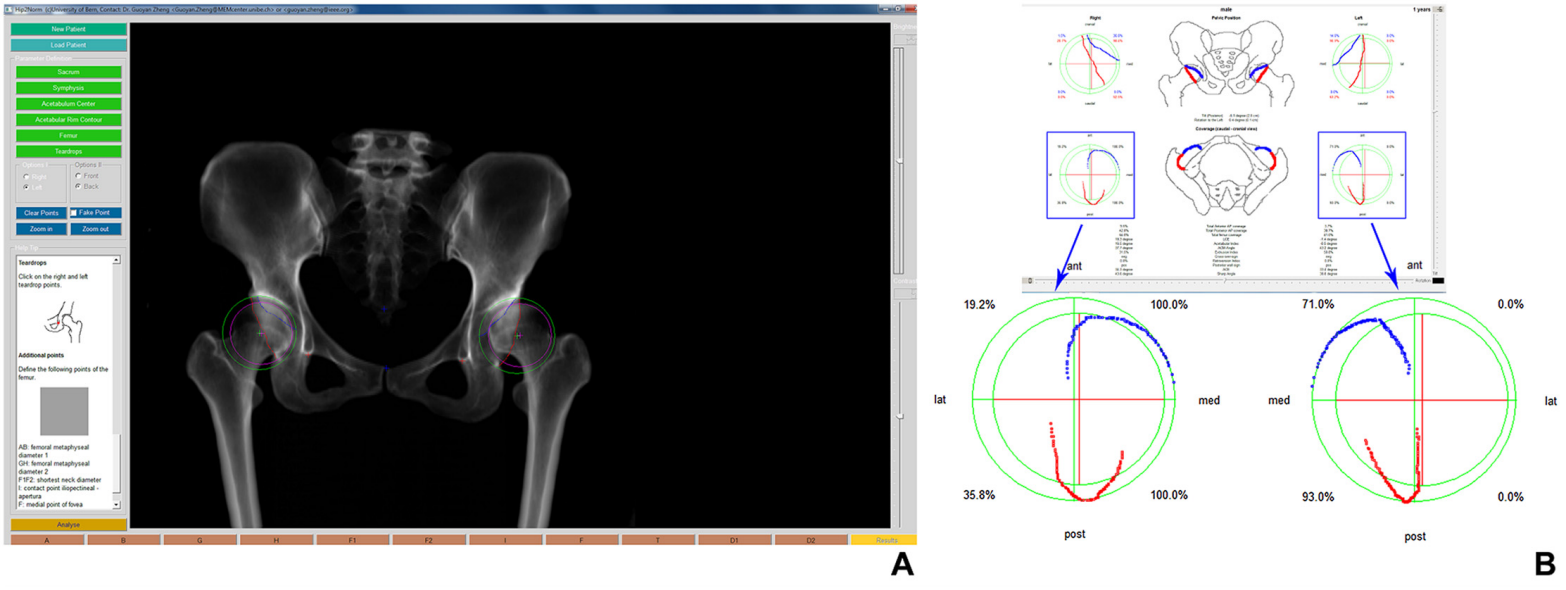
Hip^2^Norm Software. (A) Graphical user interface (left image) for digitizing landmarks for computerized evaluation of an AP radiograph of the pelvis. The inferior margins of the teardrops (red crosses), the outline of the projected anterior and posterior acetabular rim (blue and red line), and the middle of the sacrococcygeal joint (upper blue cross) and the upper border of the symphysis (lower blue cross) must be drawn manually, while the center and the radius of femoral head (pink cross) and acetabulum (green cross) are obtained by fitting a circle to three points specified by the user. (B) Anterior and posterior acetabular rims, and 2.5D measurements of FHC after neutralizing the pelvic position (right image).
